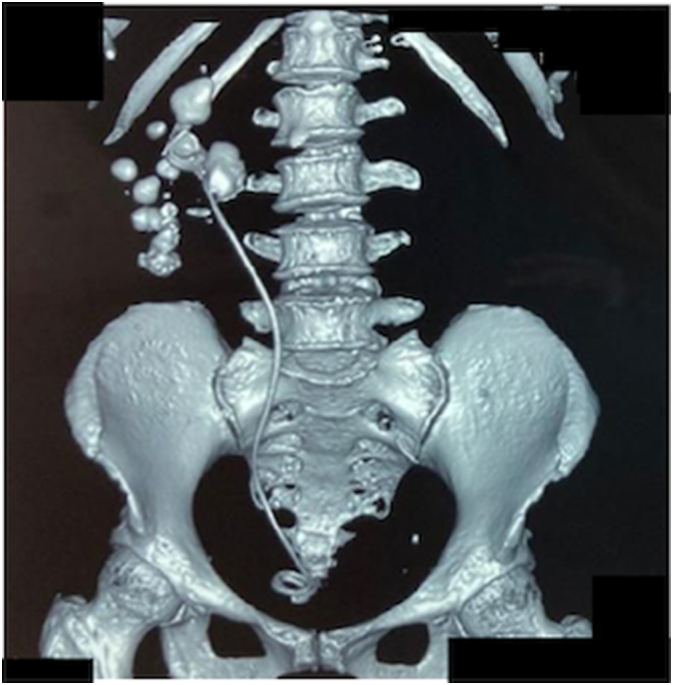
CT stonography 3D reconstruction stonography.
